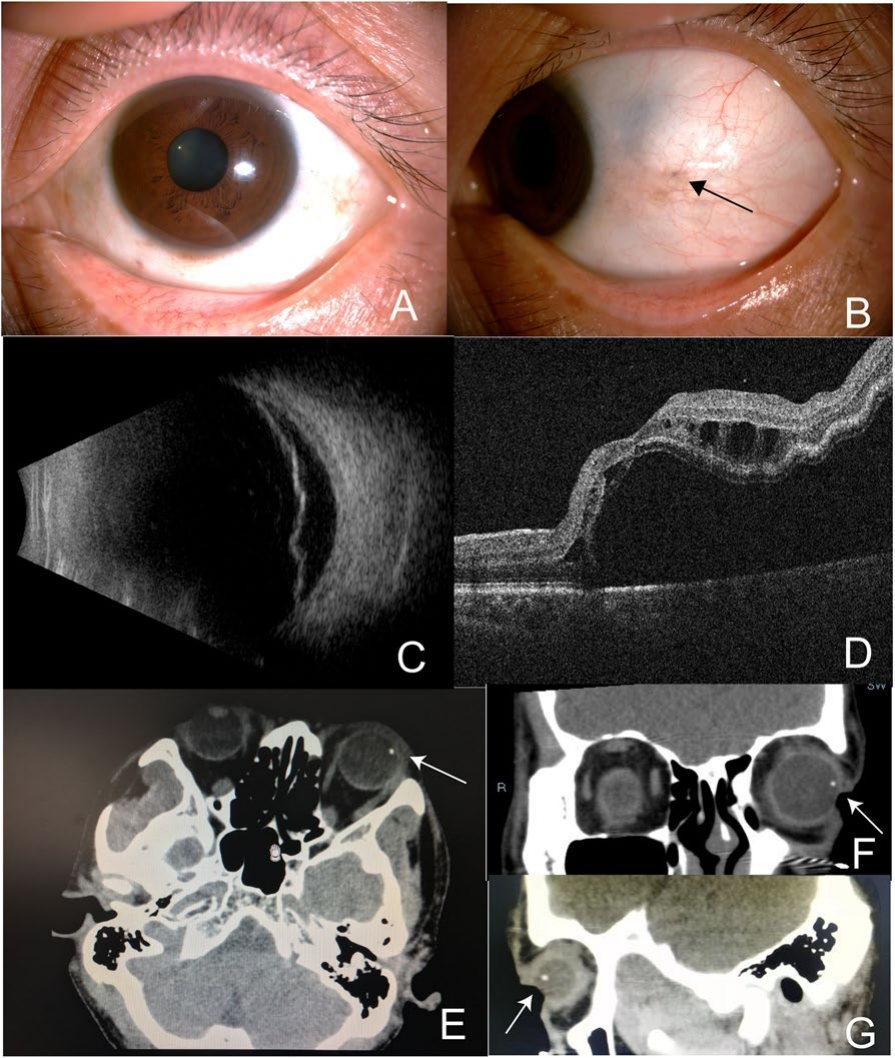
A shows that the corneal lens is clear without an obvious penetrating wound; The arrow in B shows a small patch of pigmentation in the temporal sclera, which was presumed to be the point of entry of a foreign body into the eyeball; C B-ultrasound showing retinal detachment; D OCT showing retinal detachment; the vitreous cavity was clean without pigmentation or bleeding; E horizontal axis-CT showing an intraocular high density foreign body shadow (indicated by the arrow); F coronal axis-CT scan showing an intraocular high density foreign body shadow; G sagittal axis-CT showing an intraocular high density foreign body shadow
